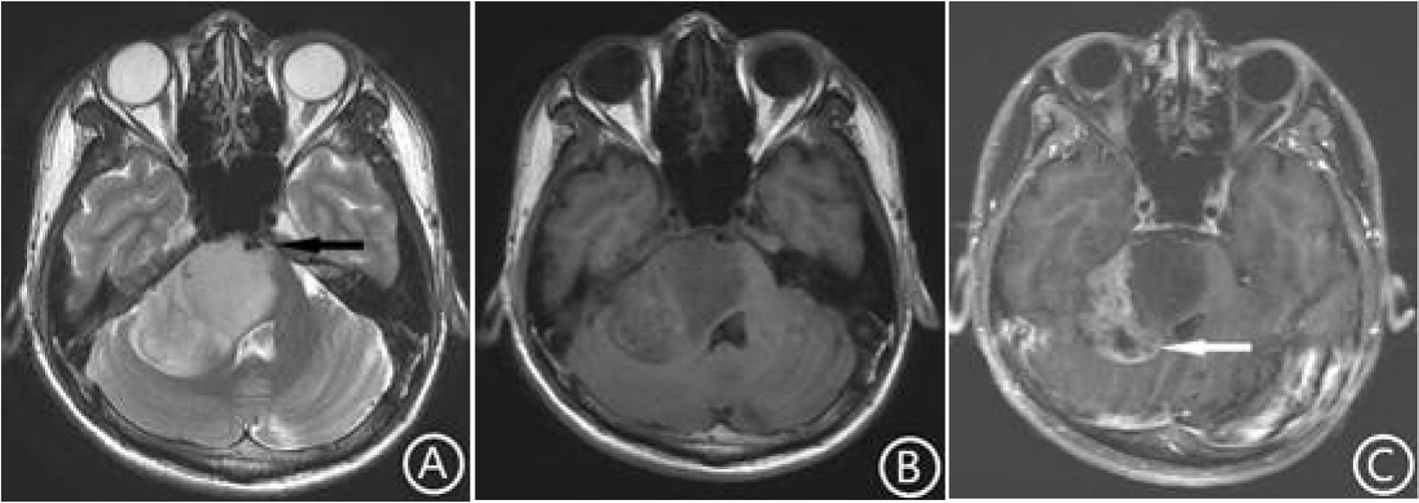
Brain MRI in patients with Diffuse midline glioma, H3 K27-altered. The lesion was located in the pons, right pontine arm and cerebellar hemisphere. The internal signal was uneven, T2WI showed a high signal (A), and T1WI showed a low signal (B). The boundary of the tumor was not clear, there was a “basilar artery wrapped sign” (A, arrow), and there was no edema around the tumor. After enhancement, the lesion showed inhomogeneous patchy enhancement and cystic necrosis (C, arrow) in the right cerebellar hemisphere and arm but no enhancement in the pons
